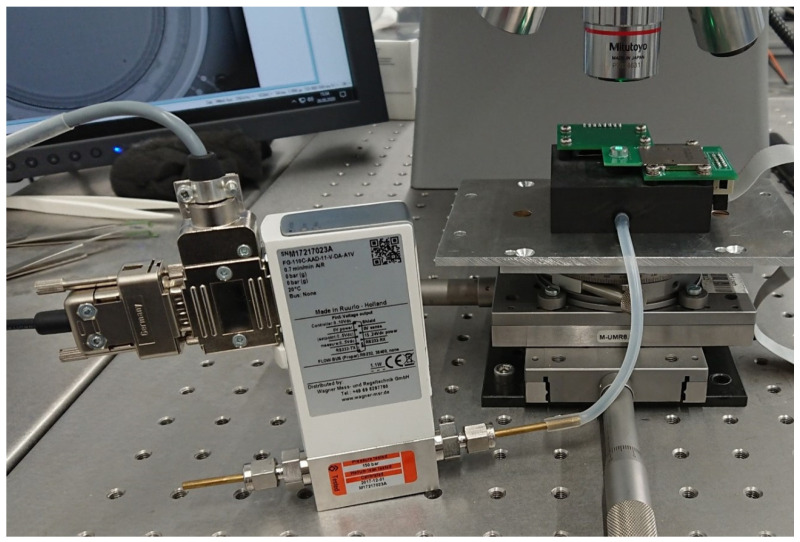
Measurement setup: Polytech MSA-500 laser Doppler vibrometer with a purpose-built test jig for prototype handling and fixation and a Bronkhorst EL-Flow Prestige mass flow meter [44].
